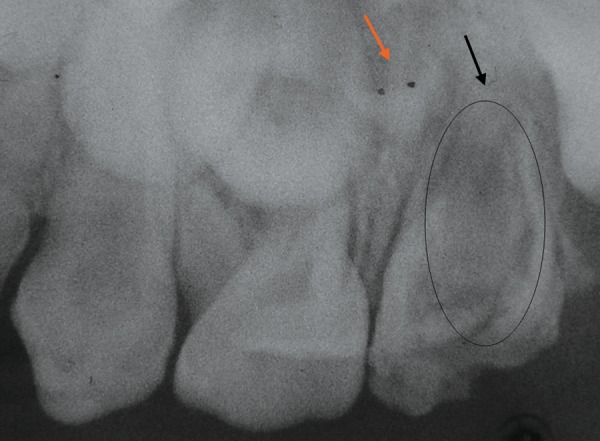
IOPA radiograph showing oval radiopacity within the crown and root portion of primary maxillary second molar (indicated by oval outline) (A) black color arrow indicating open apex of palatal root, (B) orange color arrow indicating rudimentary tooth bud of maxillary second premolar
